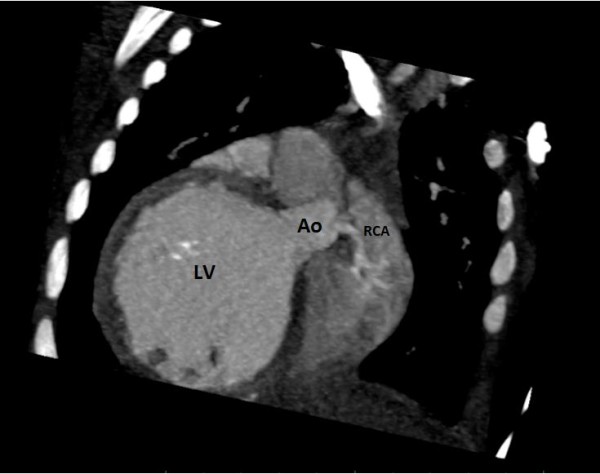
Computed tomography angiography MultiPlan. Right coronary artery emerging from aorta. Ao: Aorta. LV: Left ventricle. RCA: Right coronary artery.
